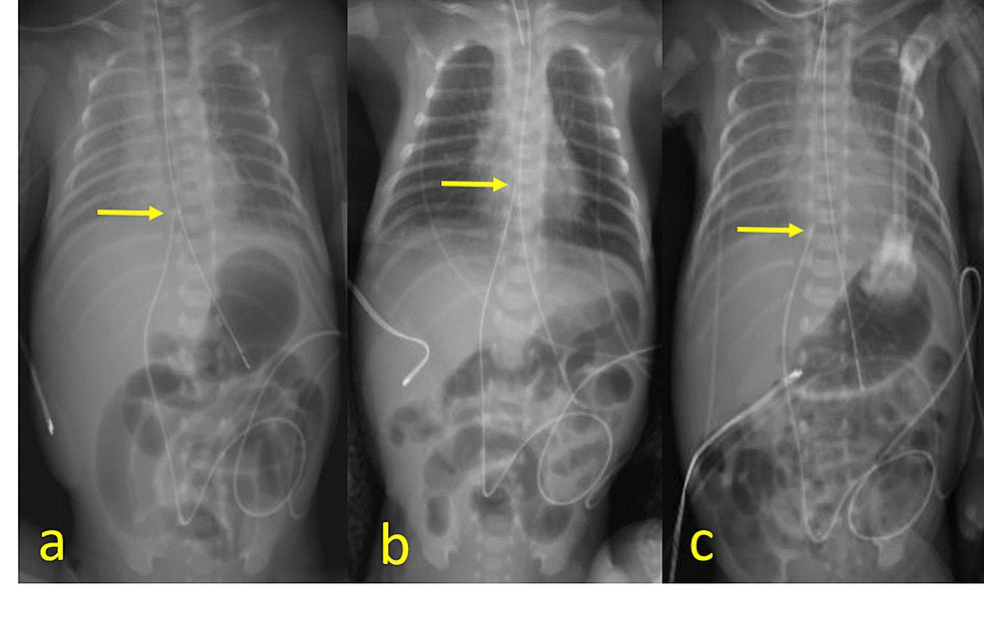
Supine portable radiographs on day 1 (a), day 2 (b), and day 5 (c) demonstrate a UVC with tip near the inferior cavoatrial junction (arrow, day 1), in the right atrium (arrow, day 2), and again near the inferior cavoatrial junction (arrow, day 5). On Day 8, a PICC was placed and the UVC was removed UVC: umbilical venous catheter; PICC: peripherally inserted central catheter
